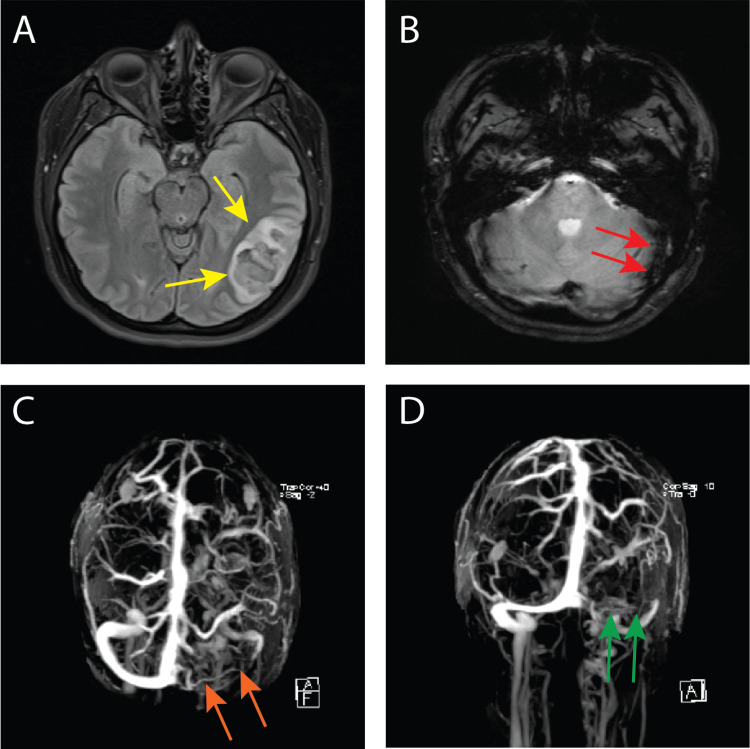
Findings on magnetic resonance imaging and magnetic resonance venography of the brain. Magnetic resonance imaging confirms the diagnosis of intraparenchymal hematoma (IPH) secondary to cerebral venous sinus thrombosis. (A) A T2 fluid-attenuated inversion recovery image is showing the left posterior temporal IPH associated with perihematomal edema (yellow arrows). (B) A gradient-related echo image is showing hypointensity involving the left transverse and sigmoid sinus (red arrows). Axial (C; orange arrows) and coronal (D; green arrows) reconstructions of magnetic resonance venography with contrast showing paucity of contrast in thrombosed sinuses.
